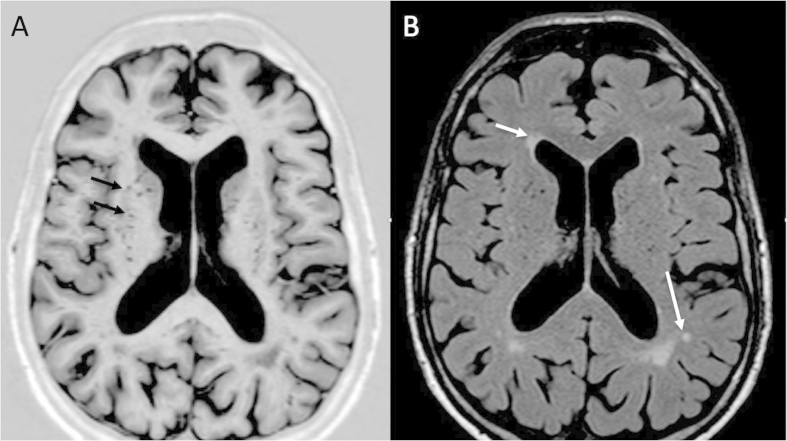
(a) T1 inversion recovery MRI of patient with POAG with multiple dilated PVS in the basal ganglia (black arrows), (b) Axial FLAIR MRI of same patient with periventricular white matter hyperintensities (white arrow) and small deep white matter hyperintensities (long white arrow).
